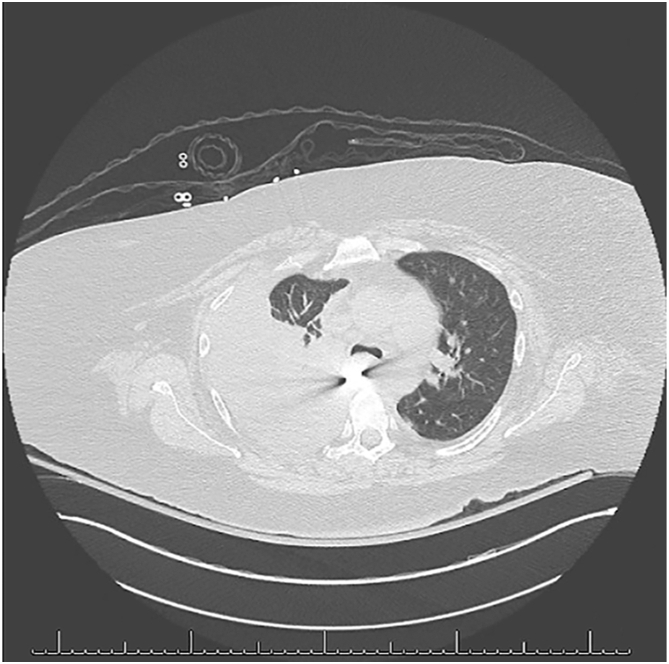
Computed tomography scan showing a large right-sided pleural effusion and concern for a radiopaque object partially intraluminal within the esophagus, extending extraluminally into the pleural space.
